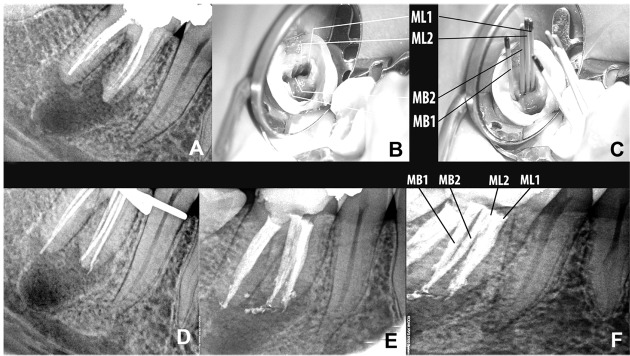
A) Preoperative radiograph B) Four root canal orifices can be observed: mesiobuccal 1 (MB1), mesiobuccal 2 (MB2), mesiolingual 1 (ML1), and mesiolingual 2 (ML2) C) Four gutta-percah master cones in four mesial canals D) Length confirmation of radiograph demonstrating four root canals in mesial root E) A radiograph after completion of retreatment. F) Postoperative radiograph showing four mesial canals in mesial root
